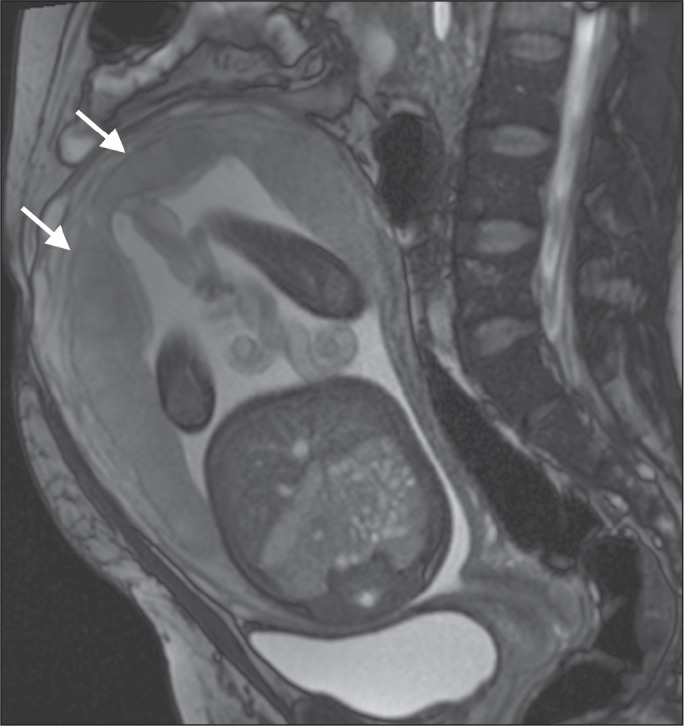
A 30-year-old patient with a normal placenta. Sagittal T2-weighted HASTE
